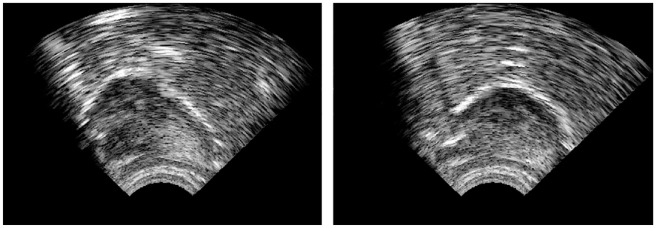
Ultrasound tongue images for /yː/ (on the left) and /uː/ (on the right). The front of the tongue is on the left side of both images.
